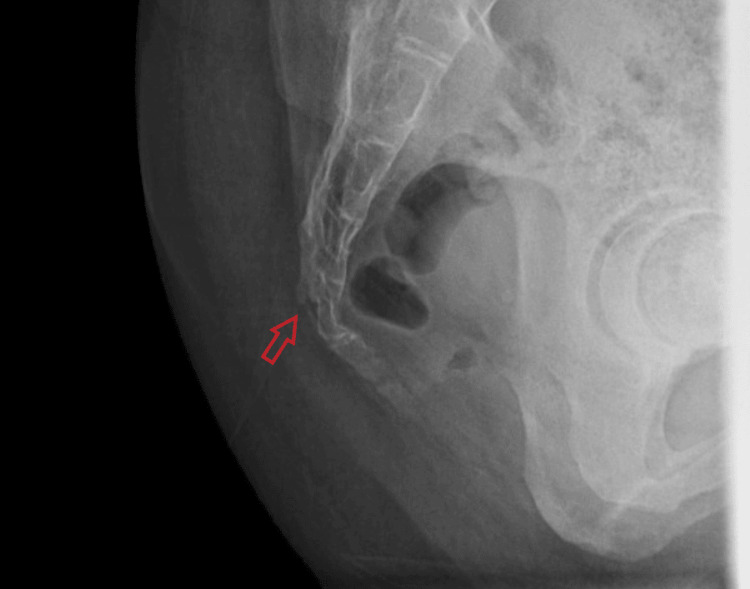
Lateral projection X-ray of the coccyx. Red arrow indicates transverse, complete, and anteriorly displaced fracture of the Cy2 segment of the coccyx.
